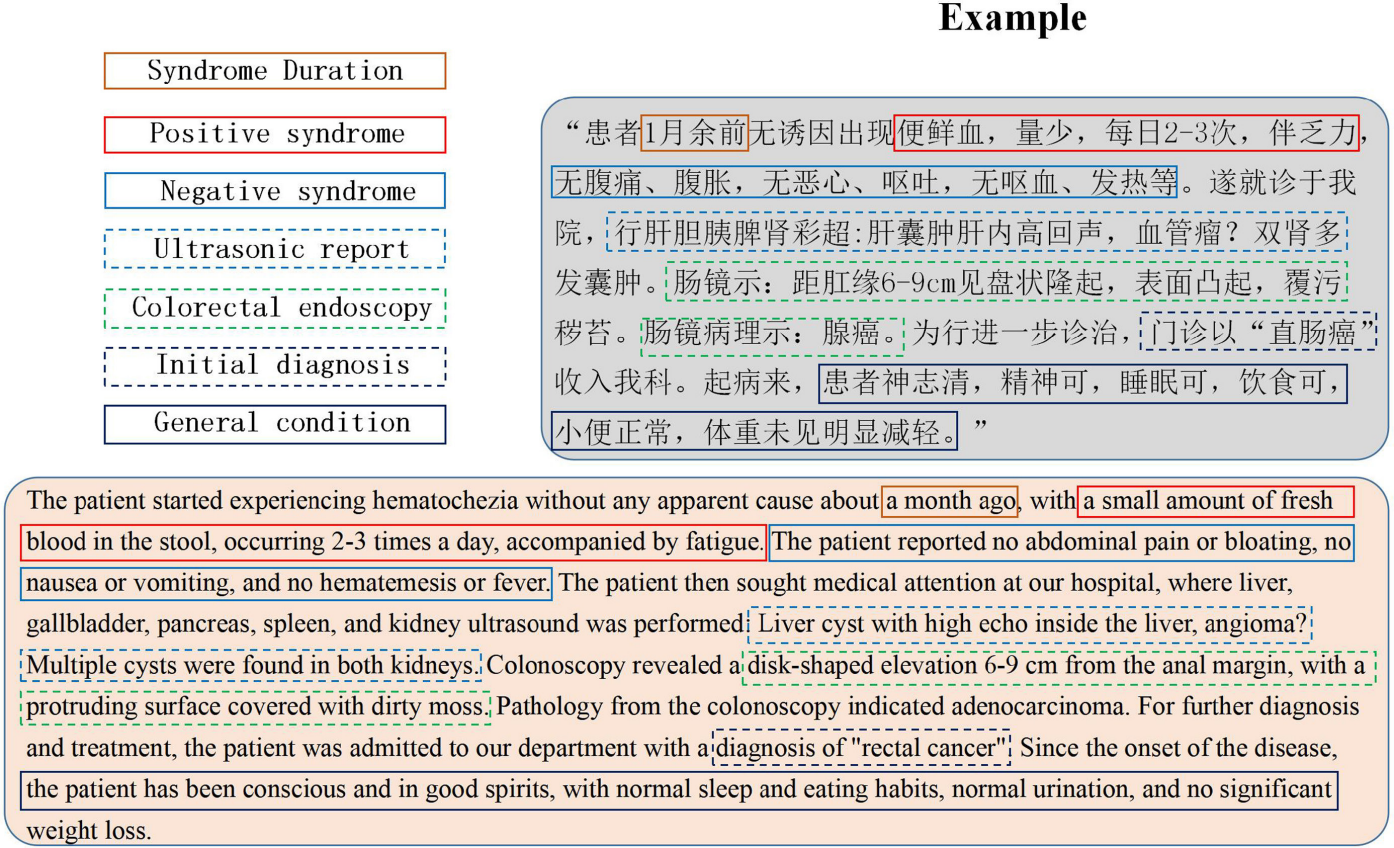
Example of an original clinical note in Chinese (upper right corner). Typically, each note provides six‐dimensional information, including positive symptoms, negative symptoms, laboratory results, imaging test results (e.g., ultrasound and colorectal endoscopy), the initial diagnosis, and a summary of the general condition. Below the original note, an English translation of the clinical note is provided.
